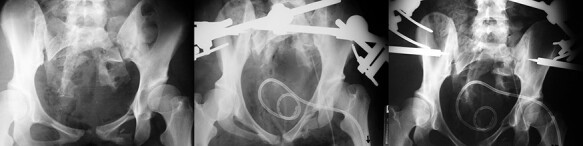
At the age of 20, patient was involved in a motor vehicle-pedestrian accident, sustaining a comminuted fracture of the sacrum and bilateral superior and inferior complicated fracture of the pubis with Morel-Lavallee lesion. X-rays of primary injury management (middle and right).
